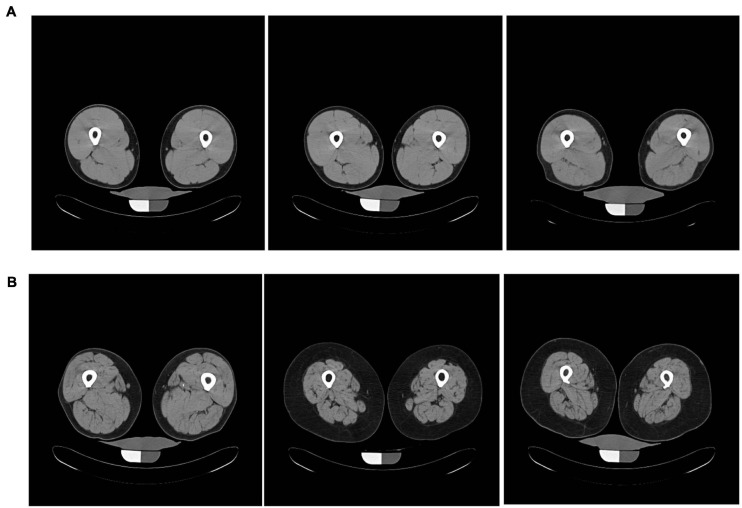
Axial mid-thigh computerized tomography images acquired from three participants with (A) low EMCL measurements (0.0031 ± 0.0002) and three participants with (B) high EMCL measurements (0.202 ± 0.085). Participants with low EMCL exhibit higher muscle CT density when compared to those with high EMCL content.
